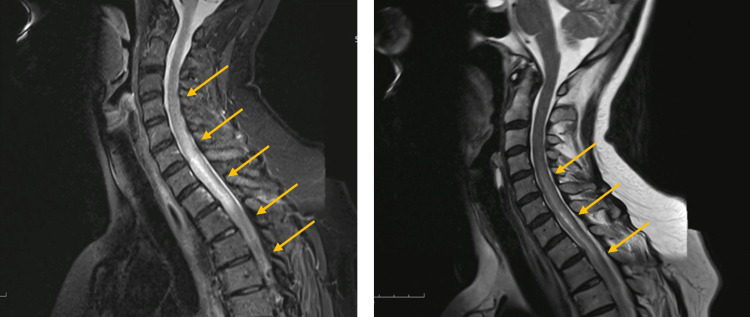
Cervical and thoracic spine MRI. MRI of the cervical and thoracic spine displaying a hyperintense signal on the short tau inversion recovery sequence on the left and gadolinium enhancement on the T2-weighted image on the right. These results suggest active inflammation in the spinal cord, which extends from the C4 to the D4 vertebrae (indicated by arrows) and are consistent with a diagnosis of longitudinally extensive transverse myelitis.
